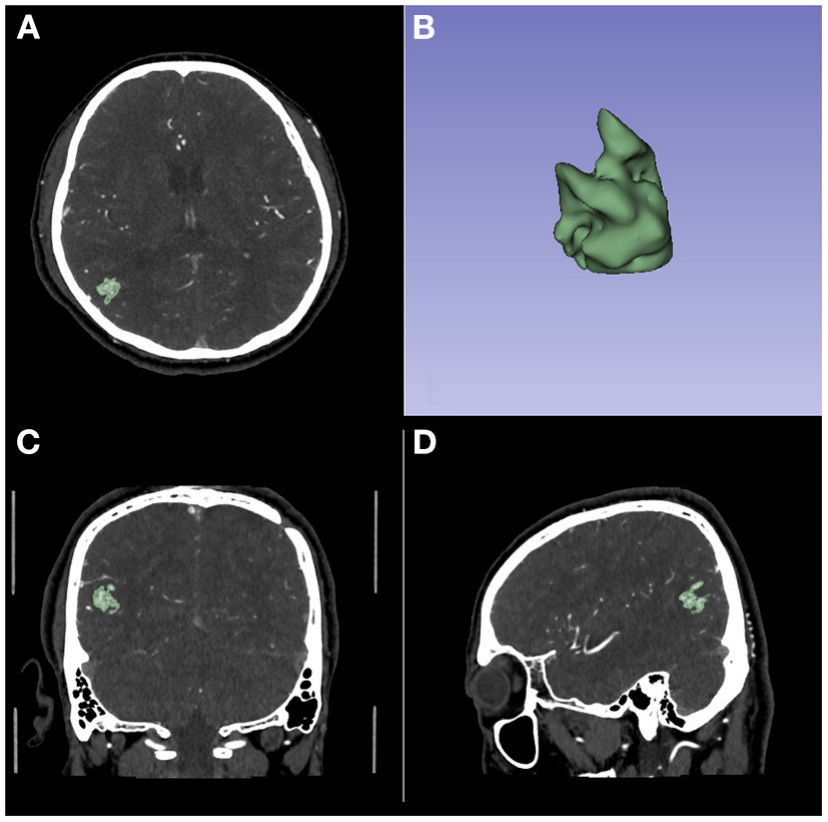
An example of 3D model building on CT Angiography image. (A) The axial image. (B) The 3D model. (C) The coronal image. (D) The sagittal image.
